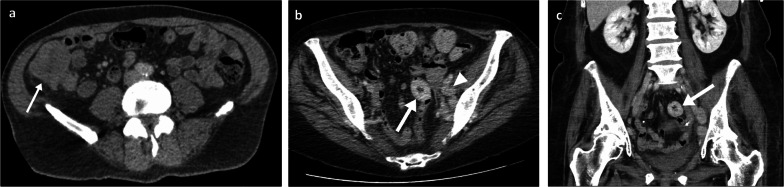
Colonic melanoma metastases. Portal phase contrast-enhanced CT axial (a) of a 71-year-old male patient with a caecal pole melanoma metastasis (arrow). Portal phase contrast-enhanced CT axial (b) and coronal (c) of 71-year-old female patient with a melanoma metastatic deposit to the sigmoid colon with circumferential thickening and enhancement (arrows) and pathological left iliac nodes (arrowhead)
